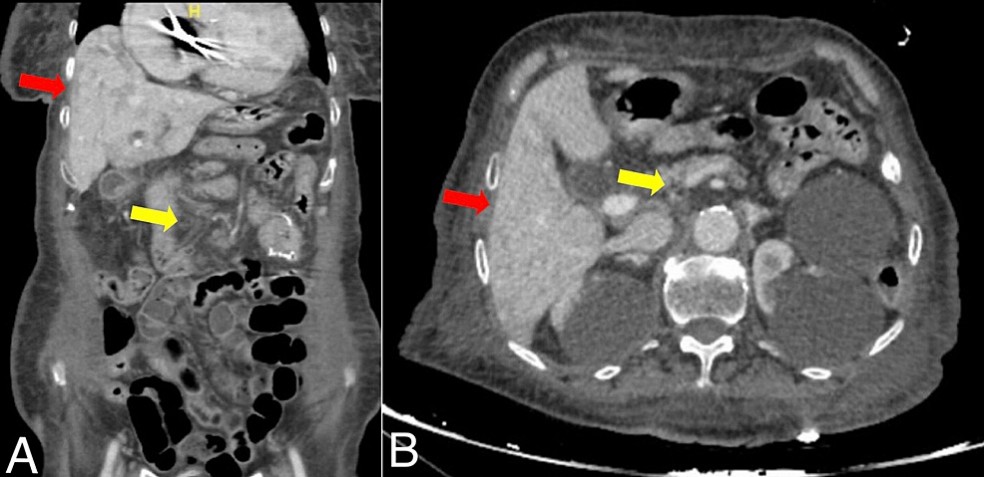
(A) Coronal and (B) sagittal views of the patient’s abdominal computed tomography revealing an enhancement of the liver (red arrows) and common bile duct dilatation (yellow arrows).
